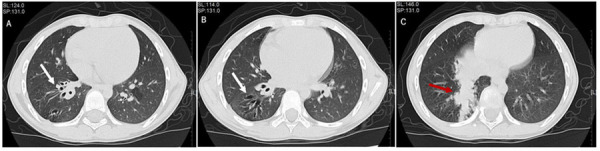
Features of a high-resolution computed tomography scan of the chest of this child. (A,B) It showed dilatation of the trachea in the right lower lobe bronchus. (C) It revealed airway mucus obstruction.
